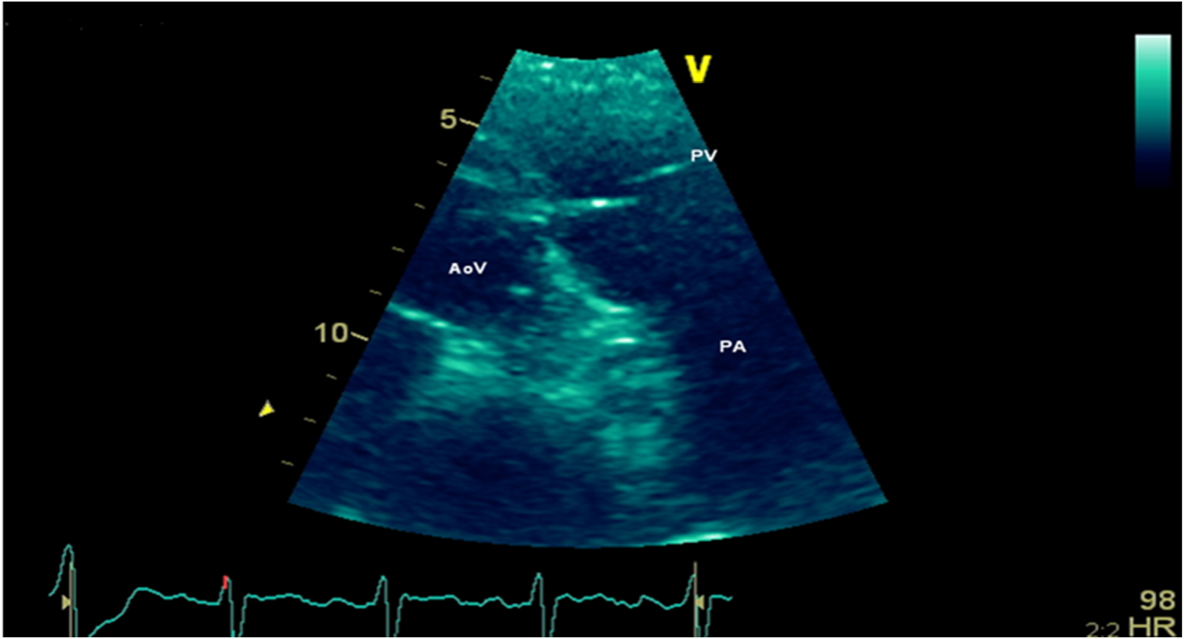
Transthoracic echocardiogram showing the pulmonary valve at discharge, after 4 weeks of empiric antibiotherapy. AoV, aortic valve; PA, pulmonary artery; PV, pulmonary valve.
